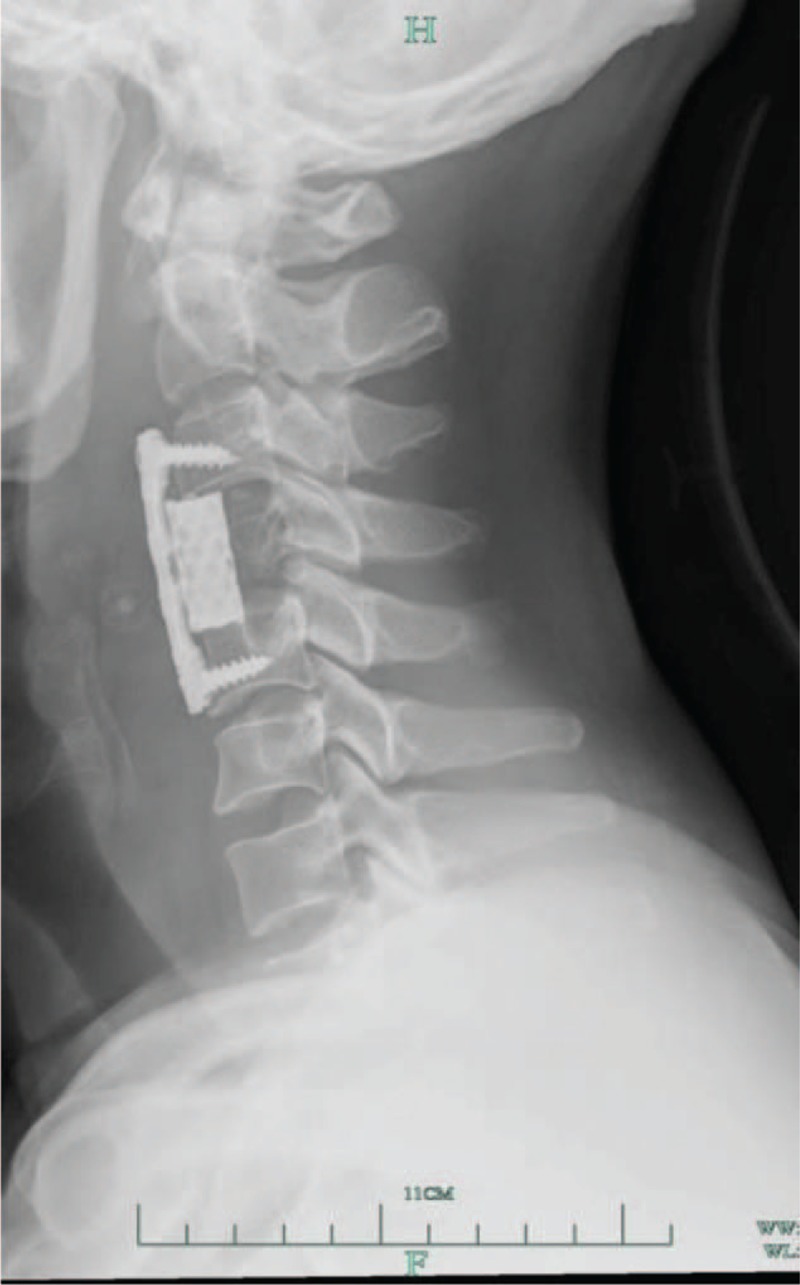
Radiographic images after surgery at C4. Lateral views are shown.
